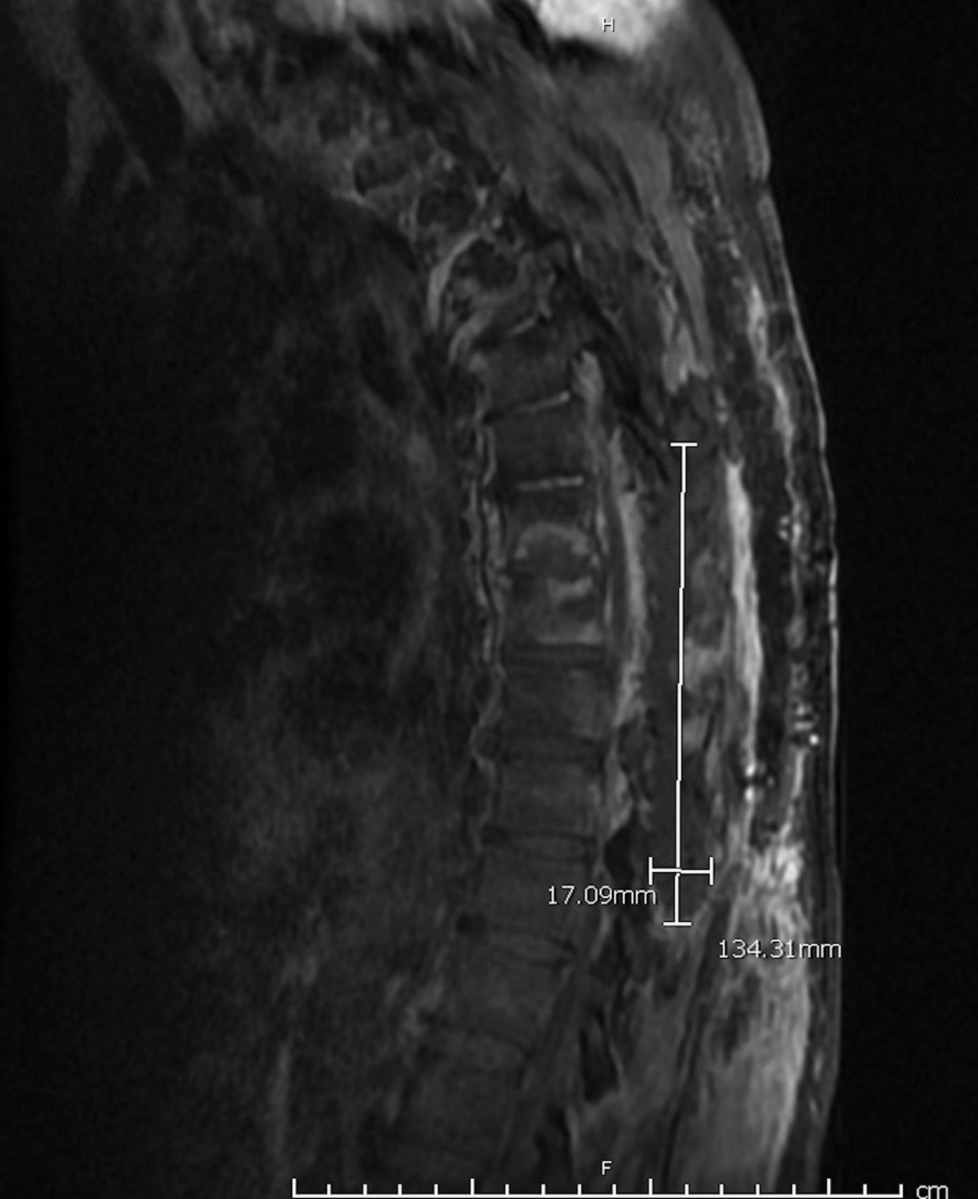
Sagittal view of thoracic spine magnetic resonance imaging (MRI). MRI of thoracic spine showing epidural phlegmon measuring approximately 13.4 cm by 1.7 cm.
